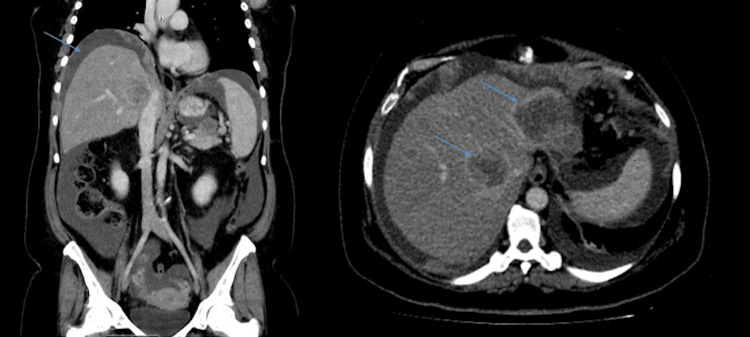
CT of the abdomen and pelvis with contrast series showing vastly diffuse metastatic disease with peritoneal carcinomatosis. The blue arrow on the right shows a moderate to large volume of ascites. Two blue arrows on the right show multiple enhancing, hepatic masses. The left hepatic lobe mass measures 4.9 cm × 4.6 cm. The right hepatic mass measures 3.8 cm × 3.7 cm. CT: computed tomography
